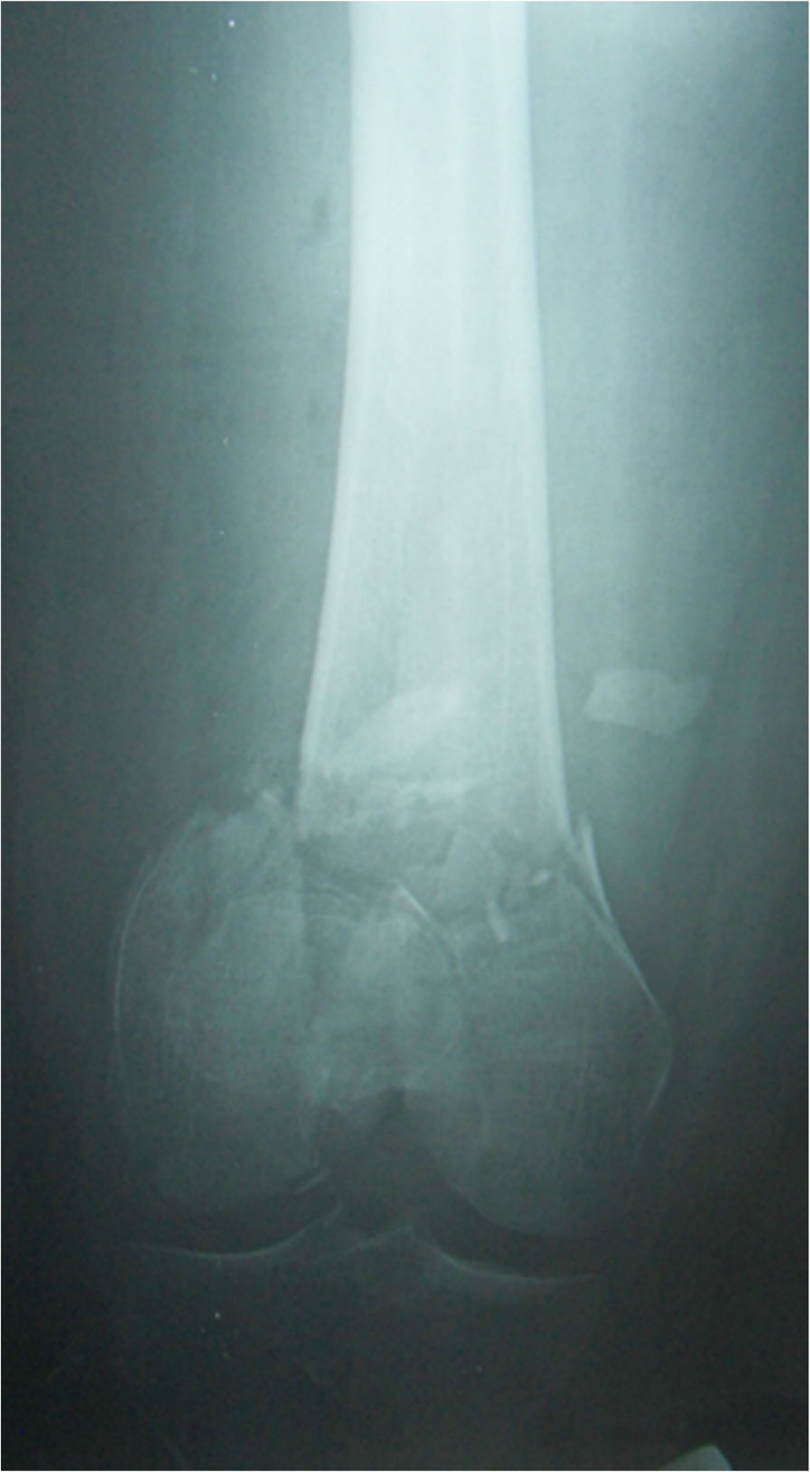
Anteroposterior radiograph of the right supra-intercondylar multifragmentary fracture of the distal femur
